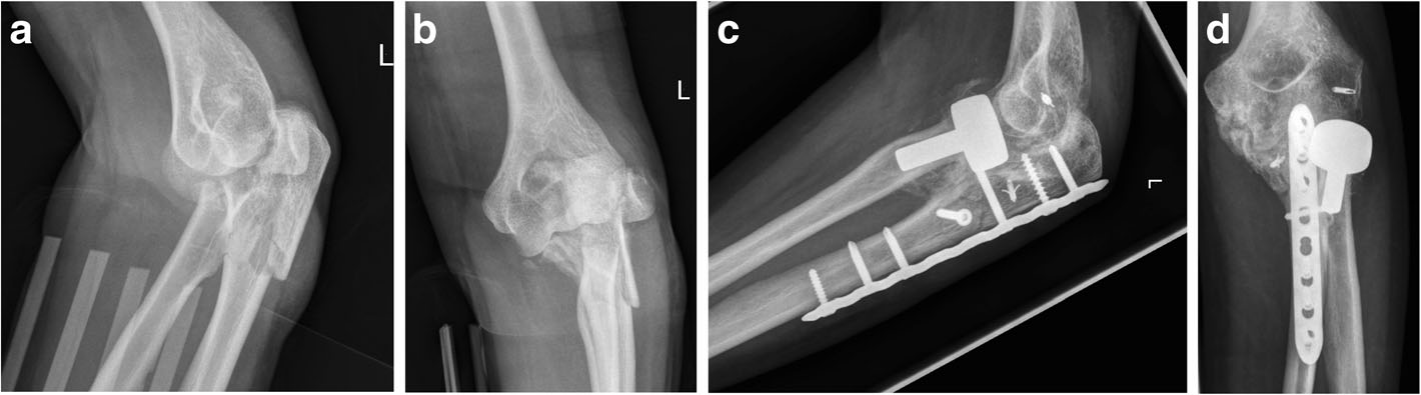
a Lateral and b anteroposterior radiographs of the left elbow of a 49-year-old woman shows a posterior Monteggia-like lesion (Bado Type II, Jupiter Type IIB), Mason Type III fracture of the radial head, and Regan and Morrey Type III coronoid fracture. c, d Six months after surgery, the patient was revised with open arthrolysis and removal of the heterotopic ossification due to a restriction of range of motion
